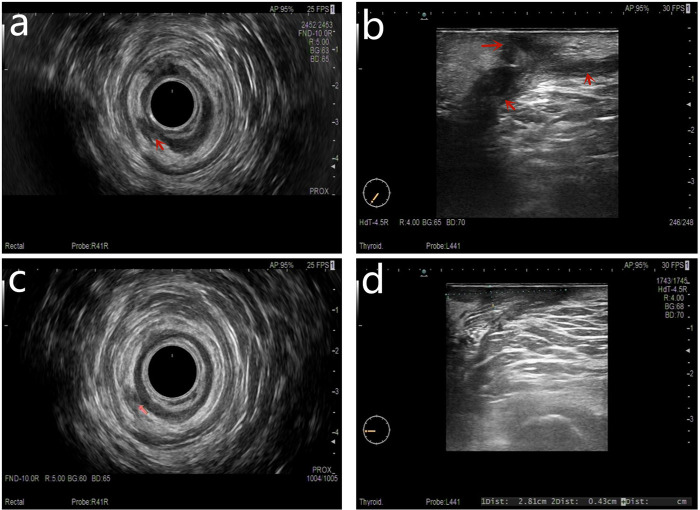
Both preoperative anal fistula and postoperative complete healing were demonstrated on a follow-up anorectal ultrasound scan. (A) Preoperation transrectal biplanar probe scans, (B) preoperation percutaneous superficial probe scans, (C) postoperation follow-up transrectal biplanar probe scans of healed fistula, and (D) postoperation follow-up percutaneous superficial probe scans of healed fistula.
